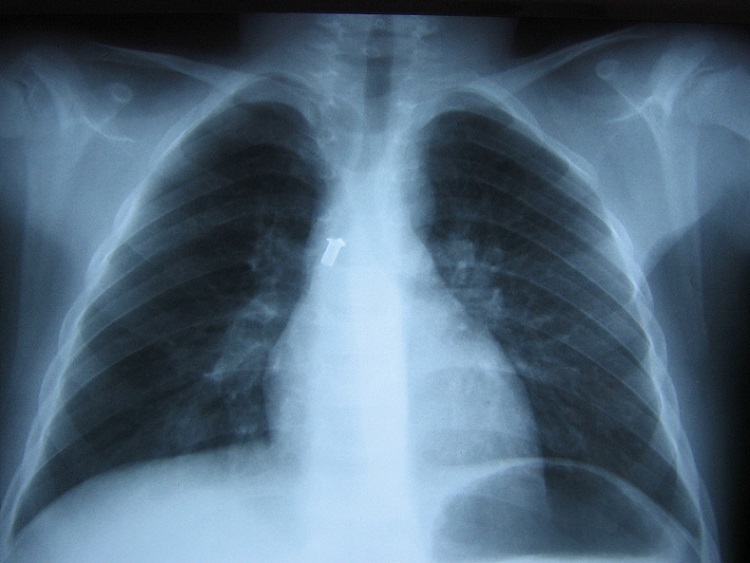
Radiographie pulmonaire objectivant un corps étranger radio opaque en regard de la bronche souche droite
